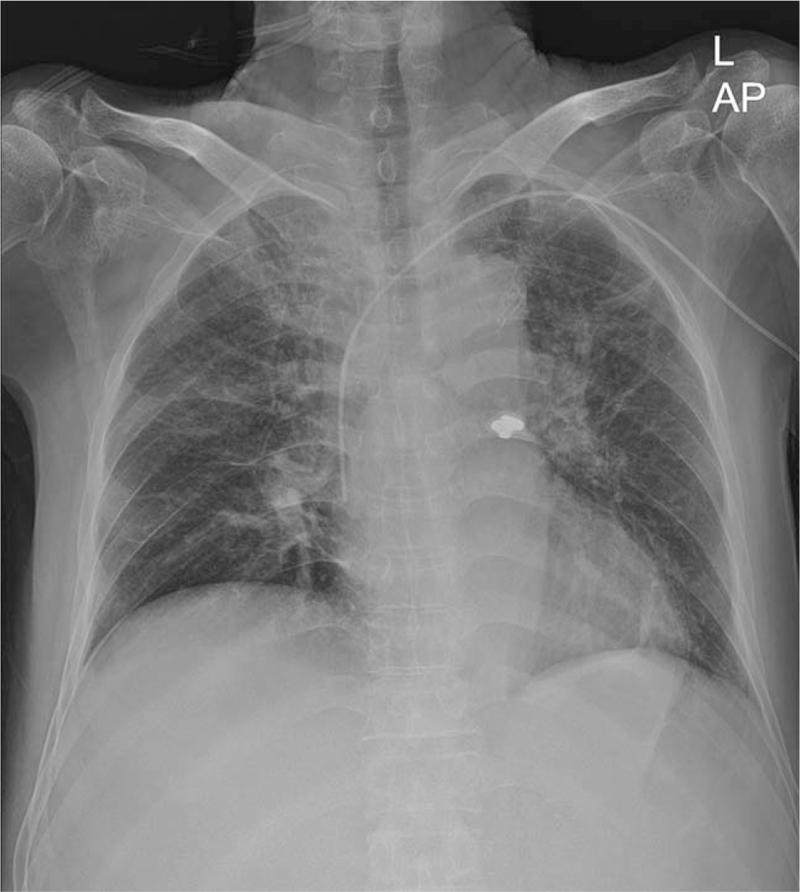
Chest x-ray showing a radio-opaque foreign material in the left main bronchial region.
